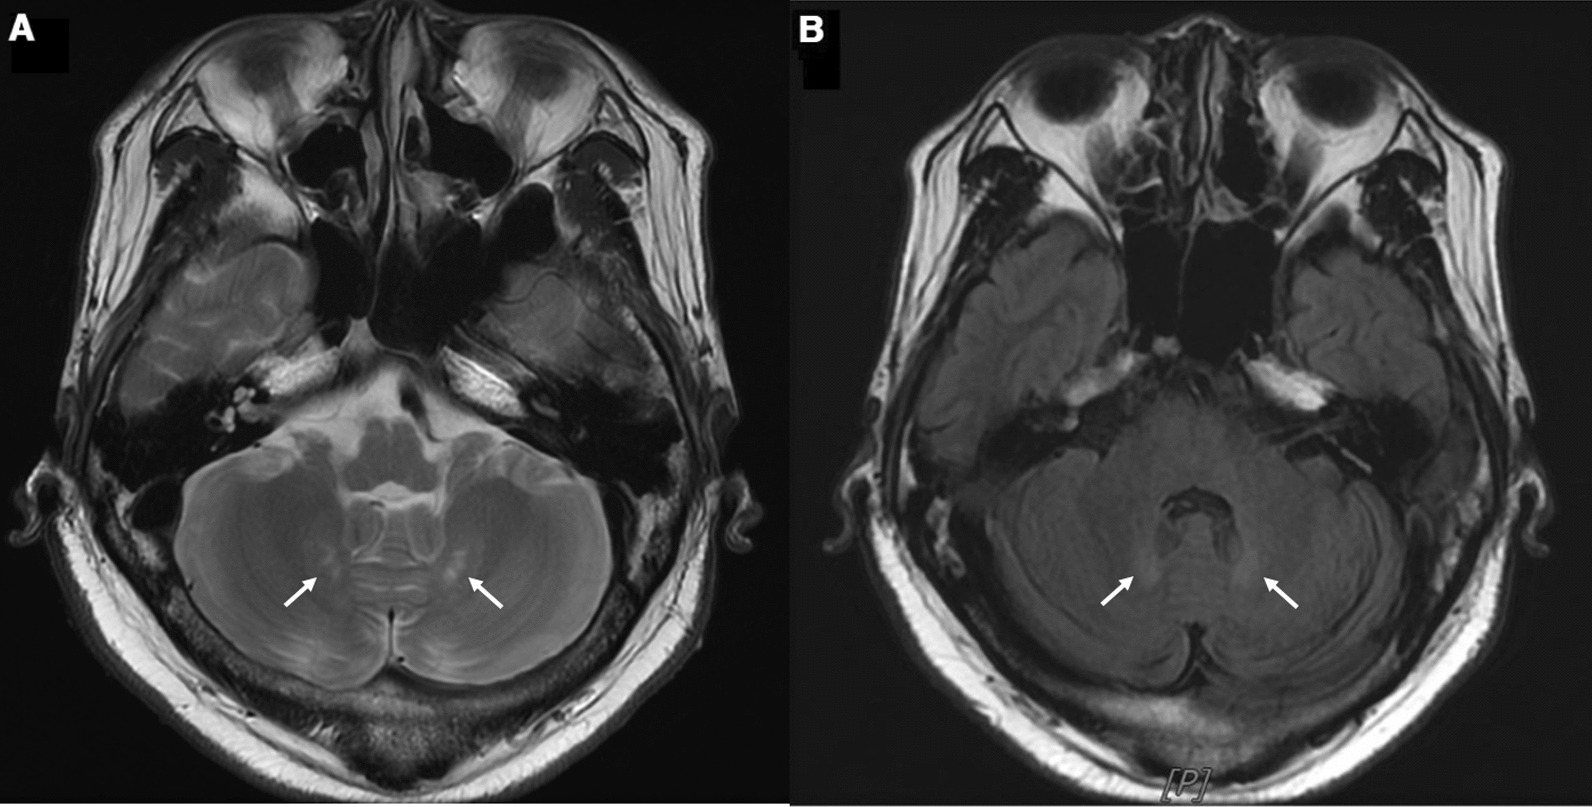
Ill-defined hyperintense lesions in bilateral cerebellar dentate nuclei were detected (white arrows) on axial T2-weighted image (A) and FLAIR image (B) of the brain
